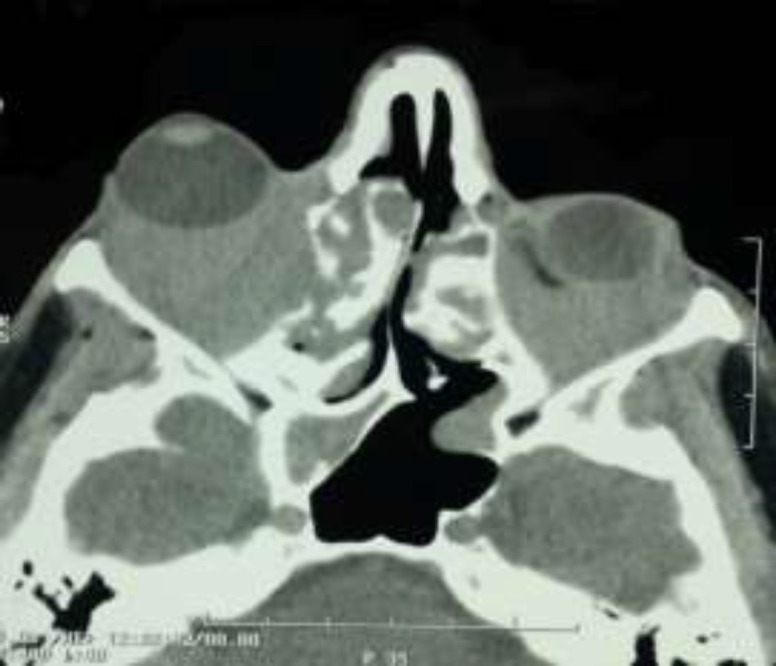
CT of PNS and orbits, showed evidence of bilateral retro-orbital enhancing masses notably in the right orbit with intraconal and extraconal extension encasement of optic nerve, and extension into the orbital apex and superior and inferior orbital
